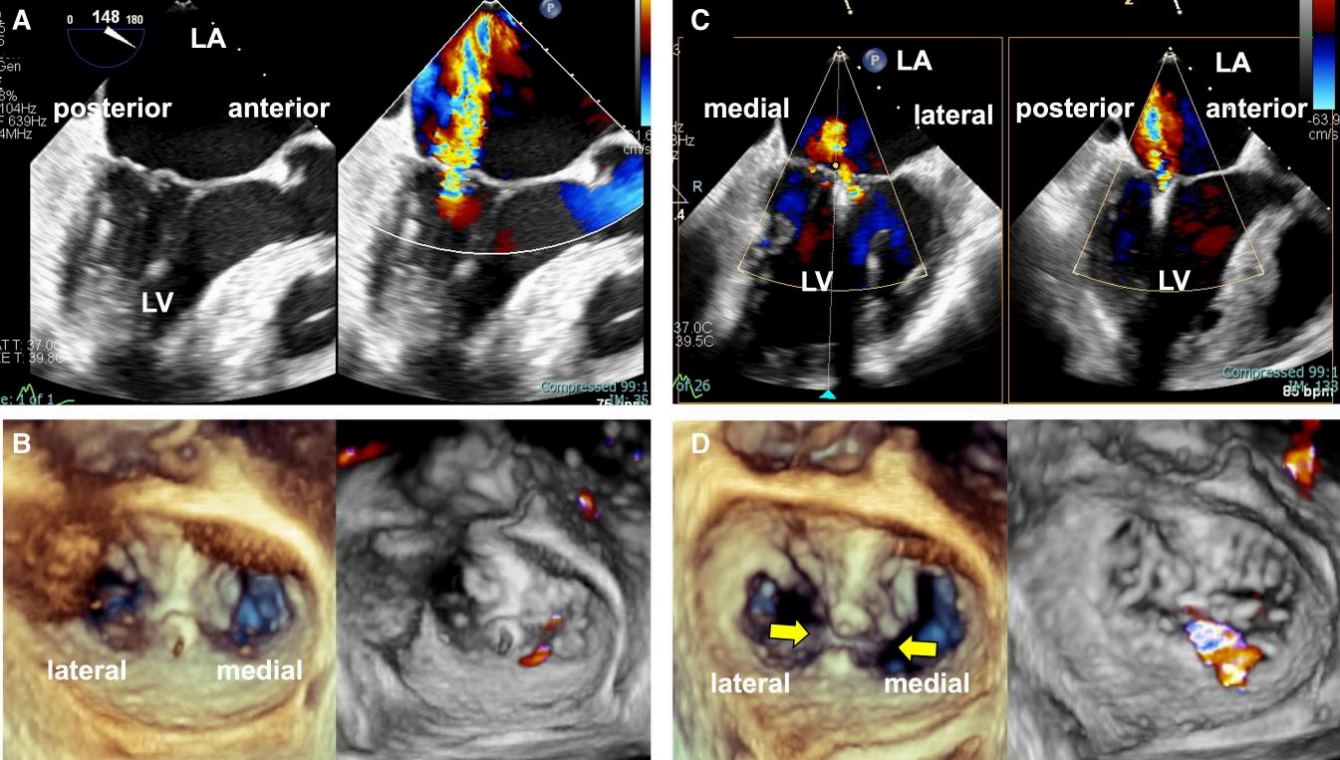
Intra-procedural transoesophageal echocardiogram images of the first transcatheter edge-to-edge mitral valve repair of Patient 1. Preprocedural transoesophageal echocardiogram showing severe mitral regurgitation (*A*). After XTW system deployment, transoesophageal echocardiogram rarely showed residual mitral regurgitation (*B*). After the XTW system was deployed, mitral regurgitation deteriorated, and aggravation of the jet was observed from the centre and medial sides of the clips (*C*). Openings between the clips were noted, which led to mitral regurgitation deterioration (*D*). LA, left atrium; LV, left ventricle.
